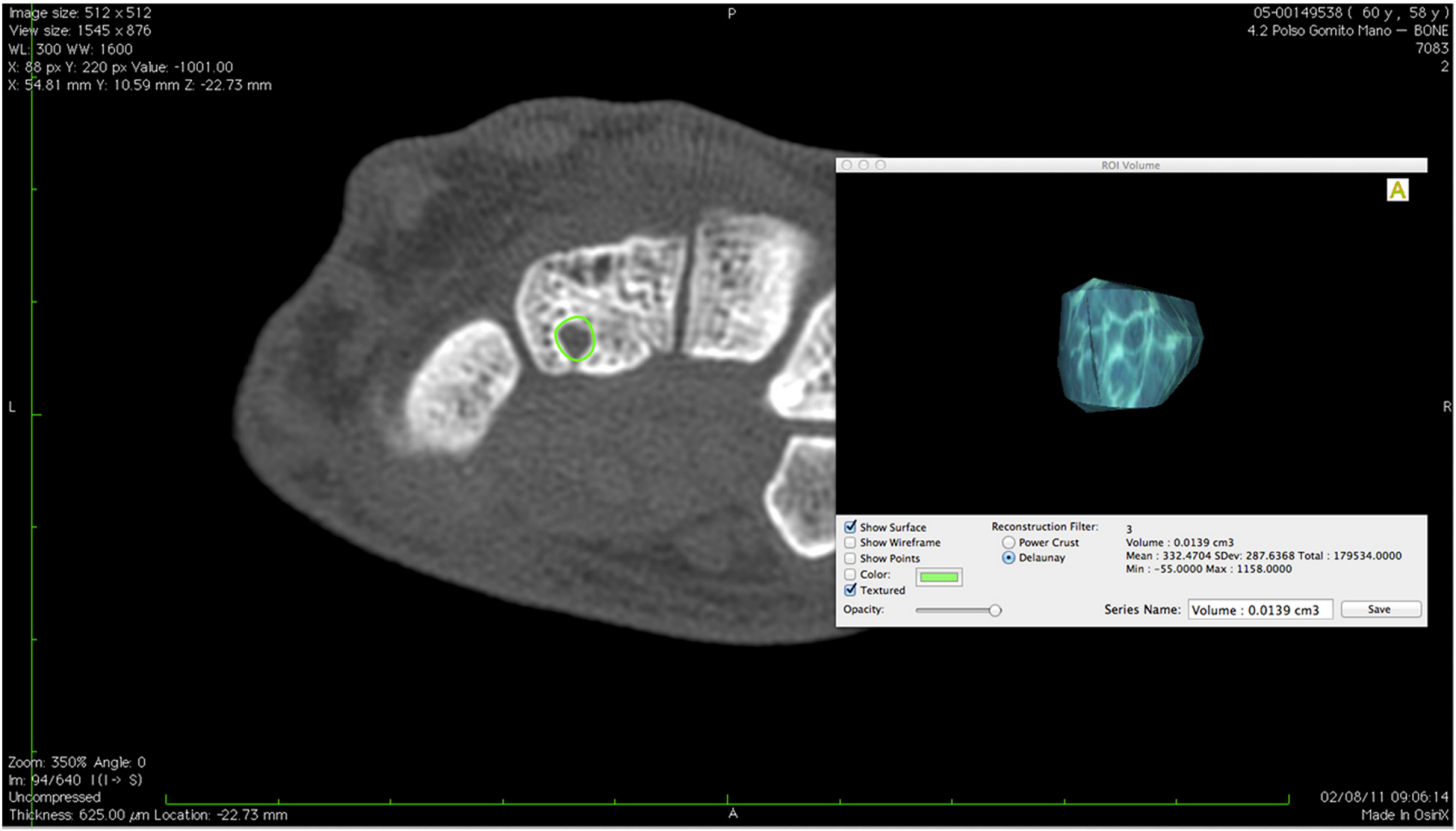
CT images of wrist *.* The erosion volume was obtained after the automatic calculation of the area of the erosion.
